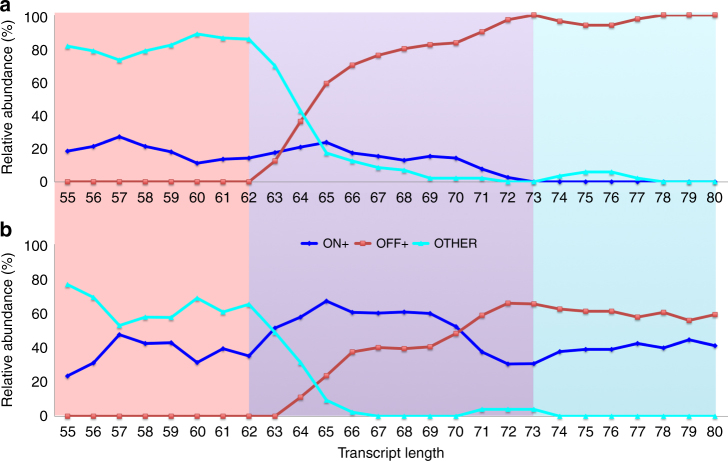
Quantitative analysis of the cotranscriptional structure landscape of *crcB* fluoride riboswitch based on SLEQ outputs. Relative abundance trajectories of ON+, OFF+, and OTHER clusters in the absence a and presence b of fluoride, as dependent on intermediate transcript length. The structures output by SLEQ were manually assigned into clusters according to whether they feature certain motifs that are key to ON and OFF structures functionality. The ON+ cluster includes structures that contain PK1. The OFF+ cluster includes structures containing P1 and the forming CT
